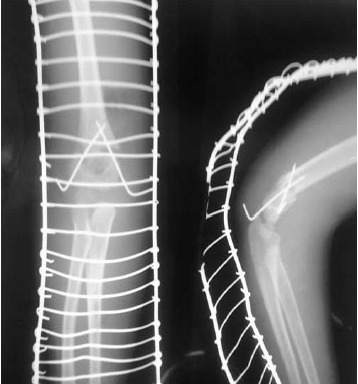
Fig. 2a: Anterior posterior radiograph at final follow up showing carrying angle F2a:
